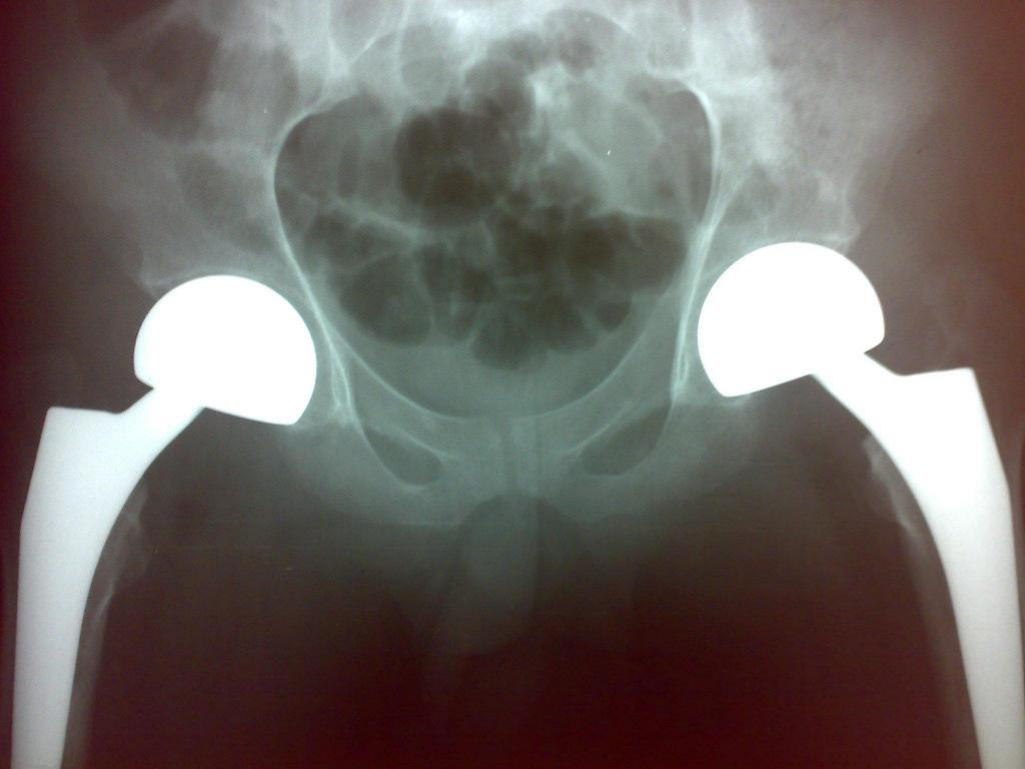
Radiograph taken after operation demonstrates replacement of left and right hip with bipolar prosthesis.
